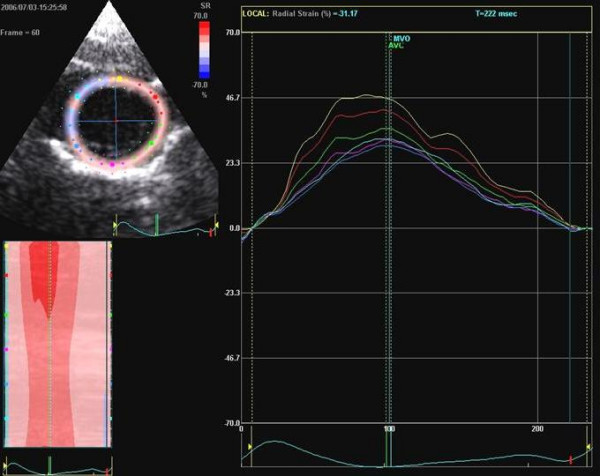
Non-Doppler 2D strain measurements of the radial deformation of the anterior and inferior wall at baseline.
